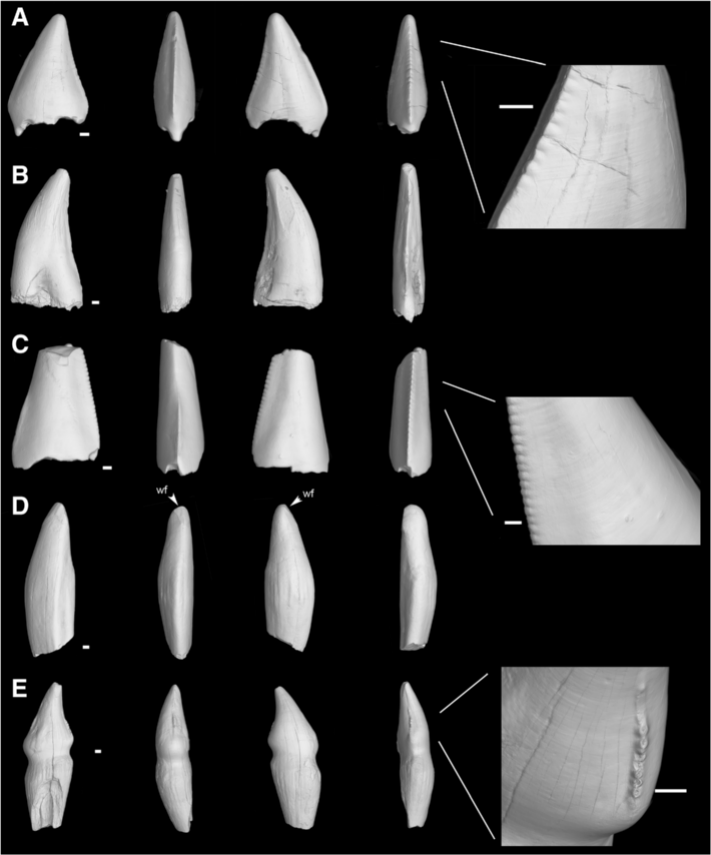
Synchrotron x-ray microtomographic views of the studied teeth of Aves and other, theropod taxa from the Late Cretaceous. a TMP 1986.030.0039, tooth crown. b TMP 1986.052.0054, tooth crown with small apical part of root preserved. c TMP 1994.031.0032, tooth crown with broken tip. d TMP 1996.012.0040, tooth with crown and two thirds of root (broken). e TMP 1989.103.0025, tooth with crown and most of root preserved (broken). Whole views are, from left to right, in a and c: labial, mesial, lingual and distal; in b, d and e: lingual, mesial, labial and distal. Magnified views show the diverse shapes and densities of serrations of the distal carina in a, c and e. wf, wear facet. All synchrotron x-ray microtomography external views. Scale bars, 0.25 mm
